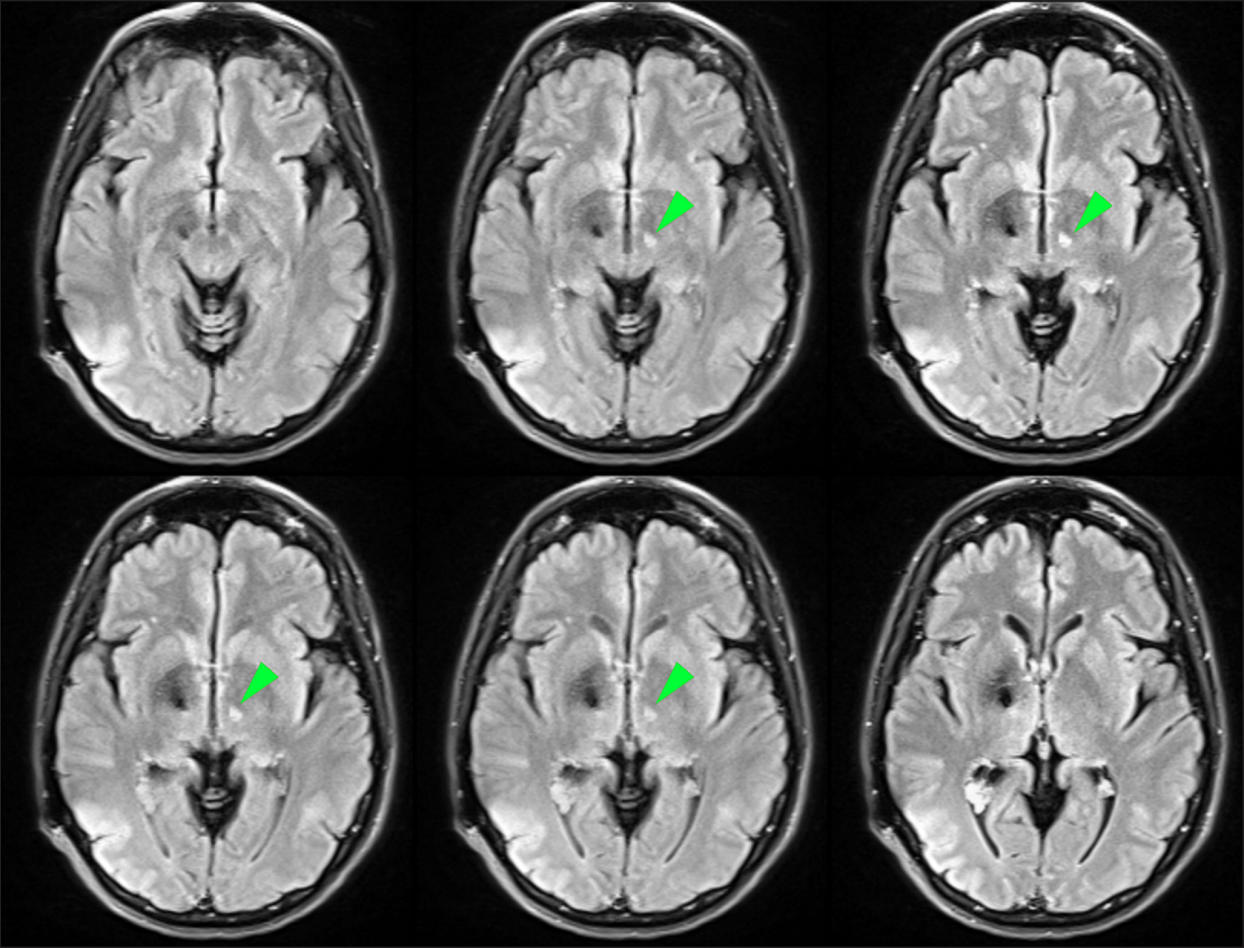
Axial sections of a brain MRI FLAIR sequence showing the lesion one-month post-procedure. The lesion can be seen on the left hemisphere as a hyperintense signal in the subthalamic region (green arrow heads).
